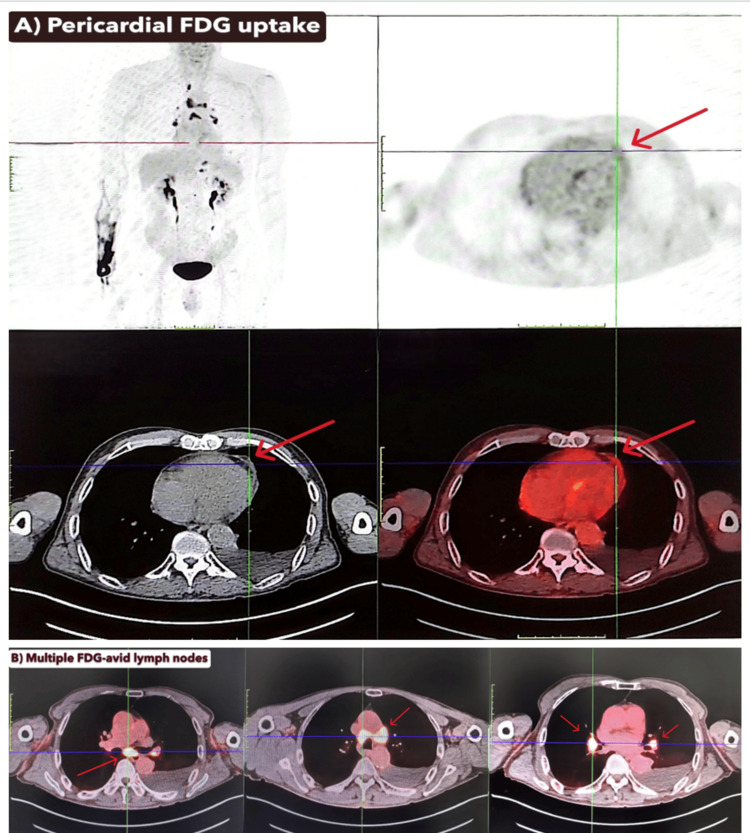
PET-CT Showing FDG-Avid Pericardium and Lymphadenopathy Whole-body F18-fluorodeoxyglucose (FDG) PET-CT demonstrated moderate FDG uptake along the left pleura and pericardium (SUVmax 3.1) (A), with prominent hypermetabolic lymph nodes in cervical, mediastinal, and abdominal stations (SUVmax up to 13.7) (B). No discrete mass lesion or primary malignancy was identified. These findings raised concern for malignancy but were later confirmed to represent a chronic inflammatory response with reactive mesothelial proliferation. SUVmax = maximum standardized uptake value
